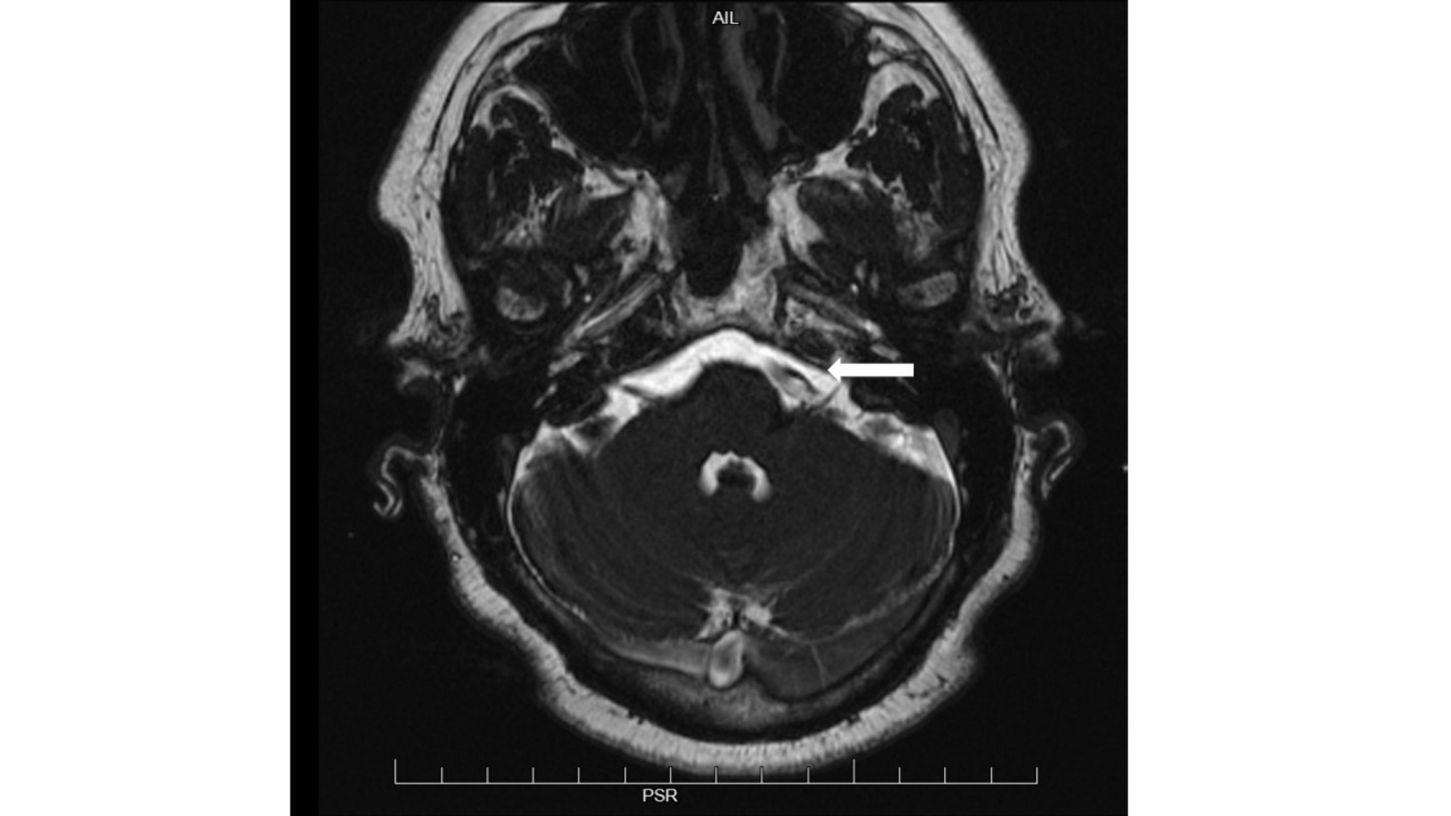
MRI of brain showing an ectatic left vertebral artery (arrow)
